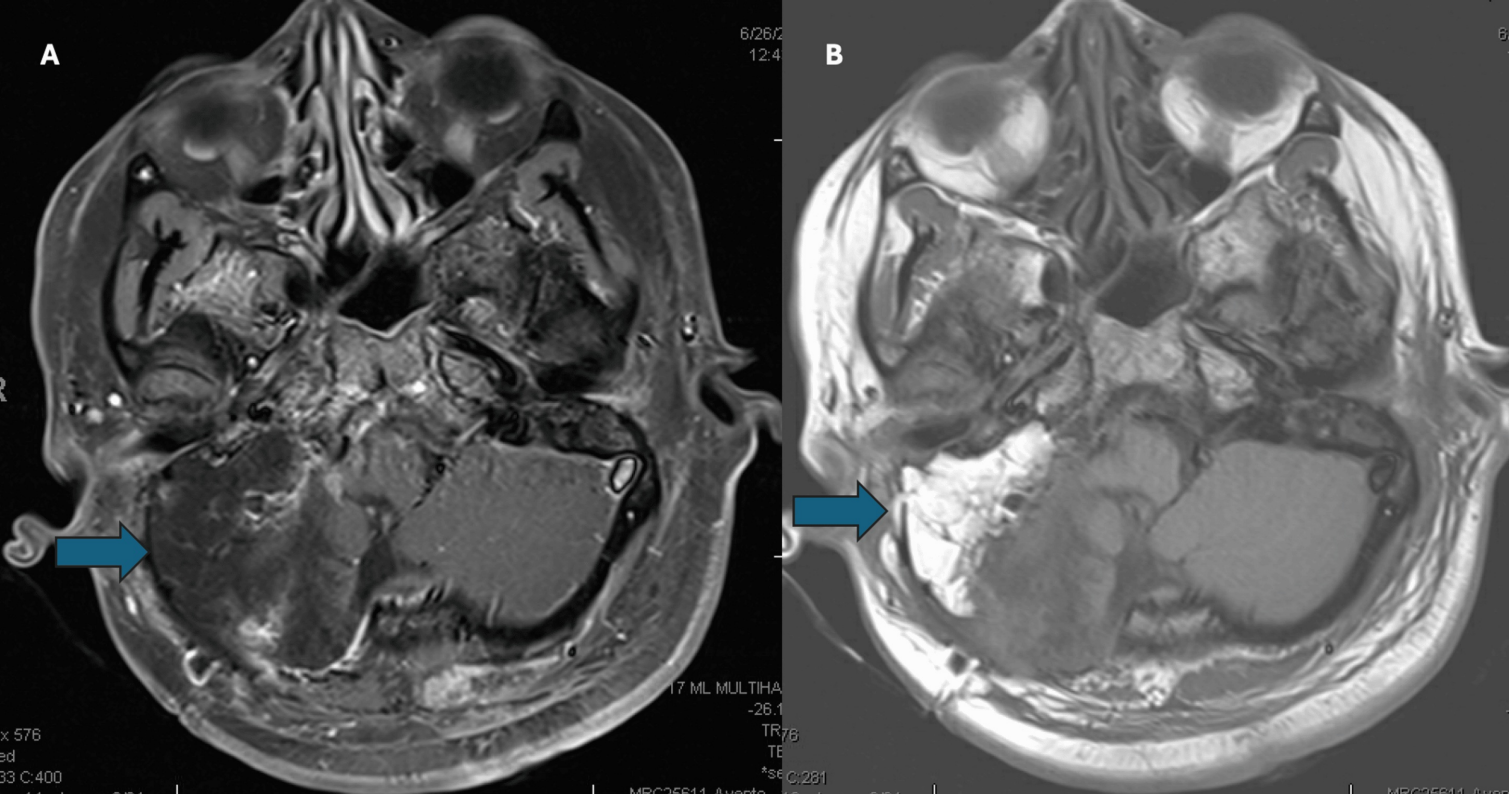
Axial brain MRI demonstrating recurrent cerebellopontine-angle meningioma in a patient with germline BAP1 mutation. (A) Axial post-contrast T1-weighted MRI showing a heterogeneously enhancing mass centered in the right cerebellopontine angle (blue arrow), with extension into the jugular foramen and hypoglossal canal, consistent with recurrent skull-base meningioma.
